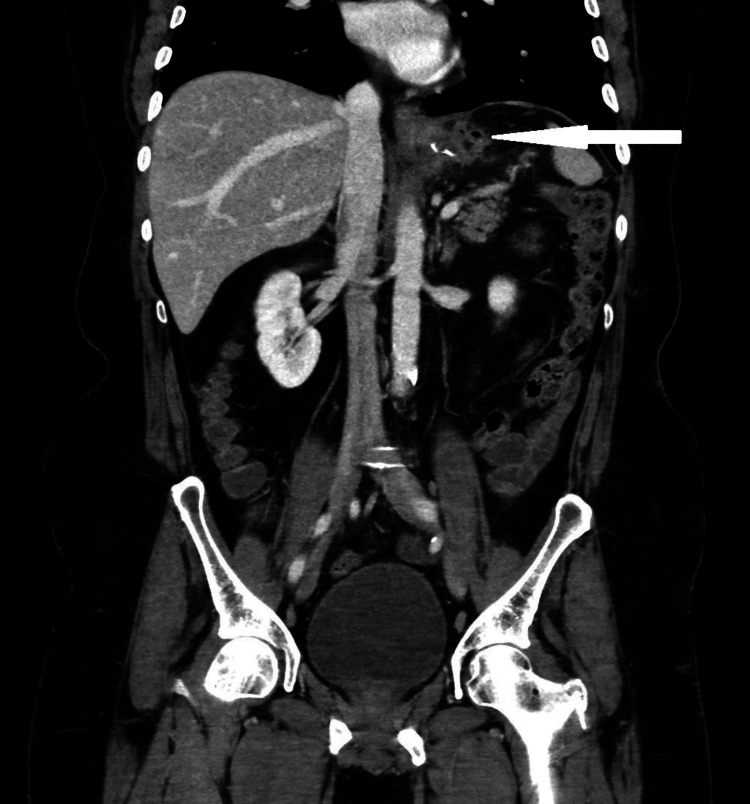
Abdominal CT scan image (coronal view) showing the fluid collection next to the upper lateral aspect of the gastric tube extending to the left subdiaphragmatic region with multiple air bubbles.
